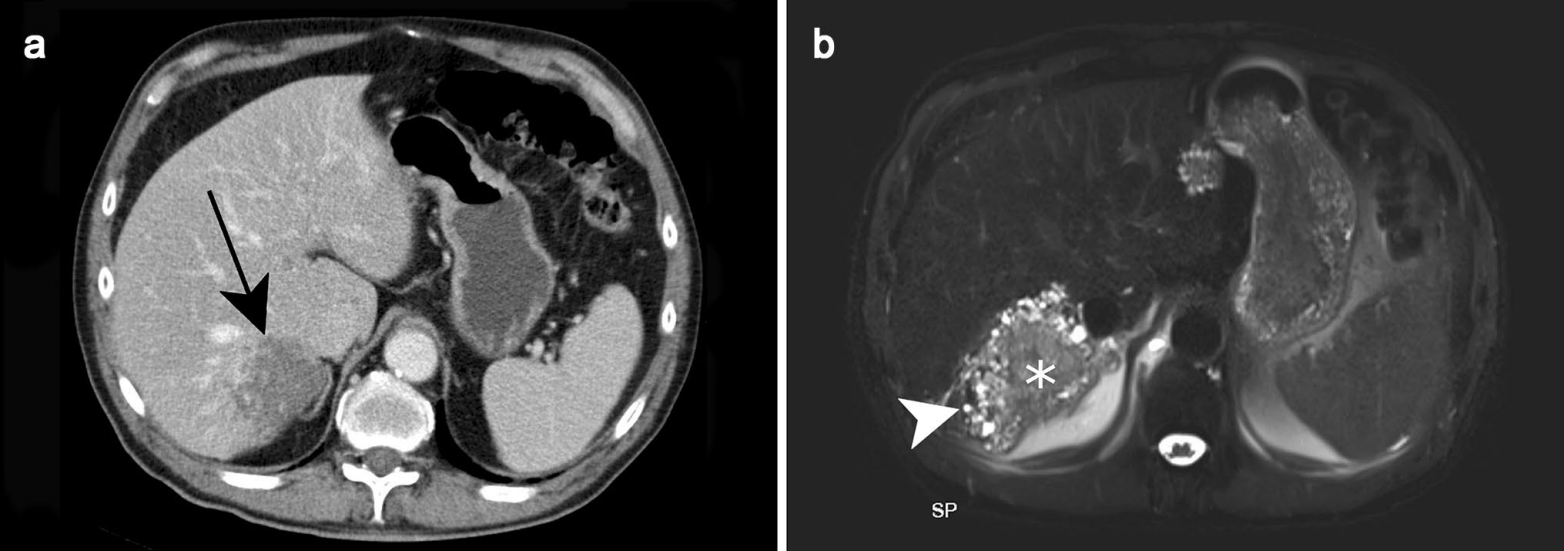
Alveolar echinococcosis incidentally detected in a 75-year-old male patient. Axial contrast-enhanced CT a shows an ill-defined subcapsular hypoattenuating lesion in segment VII of the liver (black arrow). Axial fat-suppressed T2-weighted image b further characterizes this lesion as multiple tiny cystic lesions (white arrowhead) surrounding a solid component corresponding to type 3 of alveolar echinococcosis
